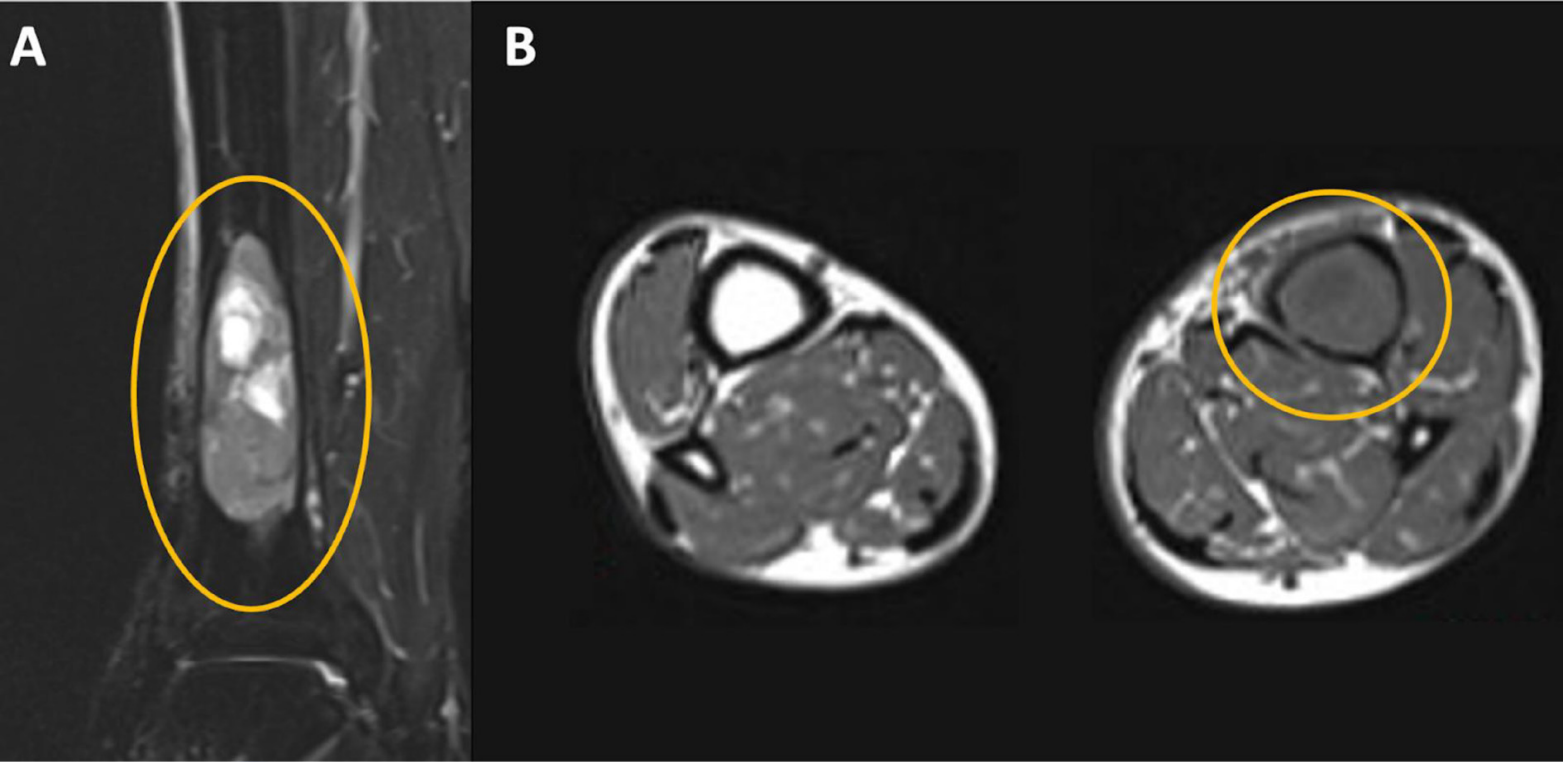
MRI images of Case 1’s neoplasm localized in the distal tibia, viewed on coronal (A) and transverse (B) axes.
